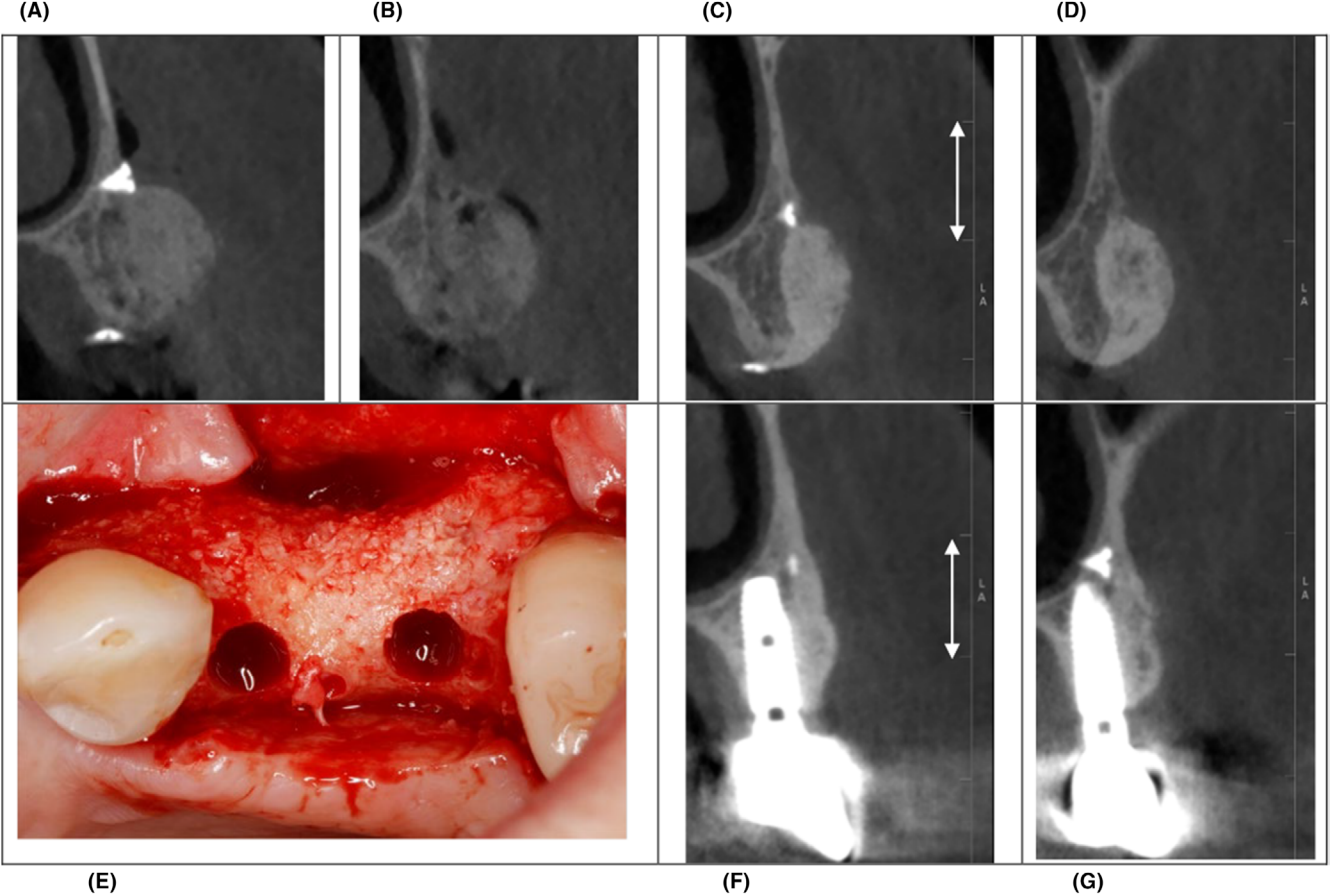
Clinical case of a staged horizontal bone augmentation via a leucocyte‐ and platelet‐rich fibrin (L‐PRF) bone block. (A and B) The patient and the referring dentist insisted on installing two implants (instead of one implant and one cantilever) to replace missing 24 and 25 teeth. Cross‐sectional cone‐beam‐computed tomography images obtained immediately after guided bone regeneration (GBR) indicated an initial alveolar ridge width of ±4 mm and a horizontal bone augmentation of 5 (24) and 7 (25) mm. (C and D) Healing after 8 months confirmed a nice bone gain with a significant increase in radiopacity and a filling of open areas (probably representing larger pieces of chapped L‐PRF). (E): Re‐entry 8 months after horizontal GBR showed a dense bone, without loose Bio‐Oss particles, and well‐vascularized osteotomies. (F and G) Cross‐sectional CBCT images in the middle of each implant, taken after 1.5 years of implant loading. Over time, early and late graft resorption is observed, a standard observation after horizontal GBR (for details, see Quirynen et al.^11^).
